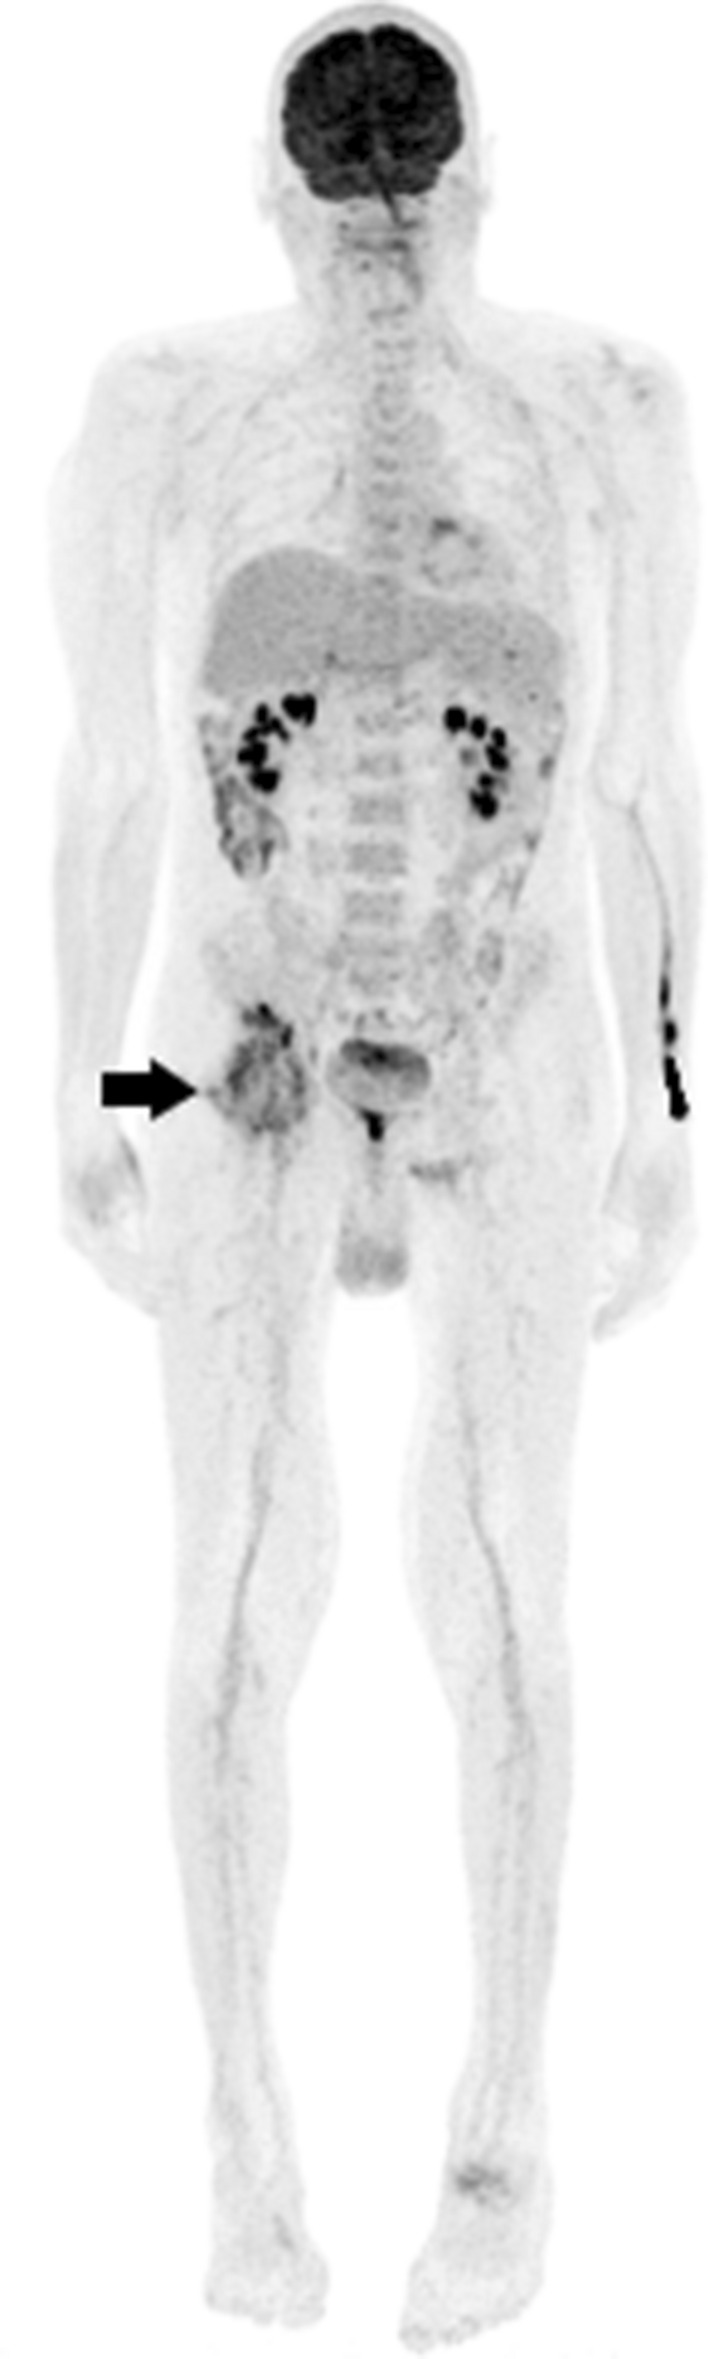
Patient no. 172, 60-year-old male, met the FUO criteria. F-18 FDG PET/CT whole-body imaging shows pathological uptake ad the right hip joint (arrow). Previous clinical examinations suggested activated osteoarthrosis. Biopsy and blood cultures performed after F-18 FDG PET/CT confirmed infective coxitis caused by Staphylococcus aureus. Patient fully recovered after prolonged i.v. antibiotic treatment.
